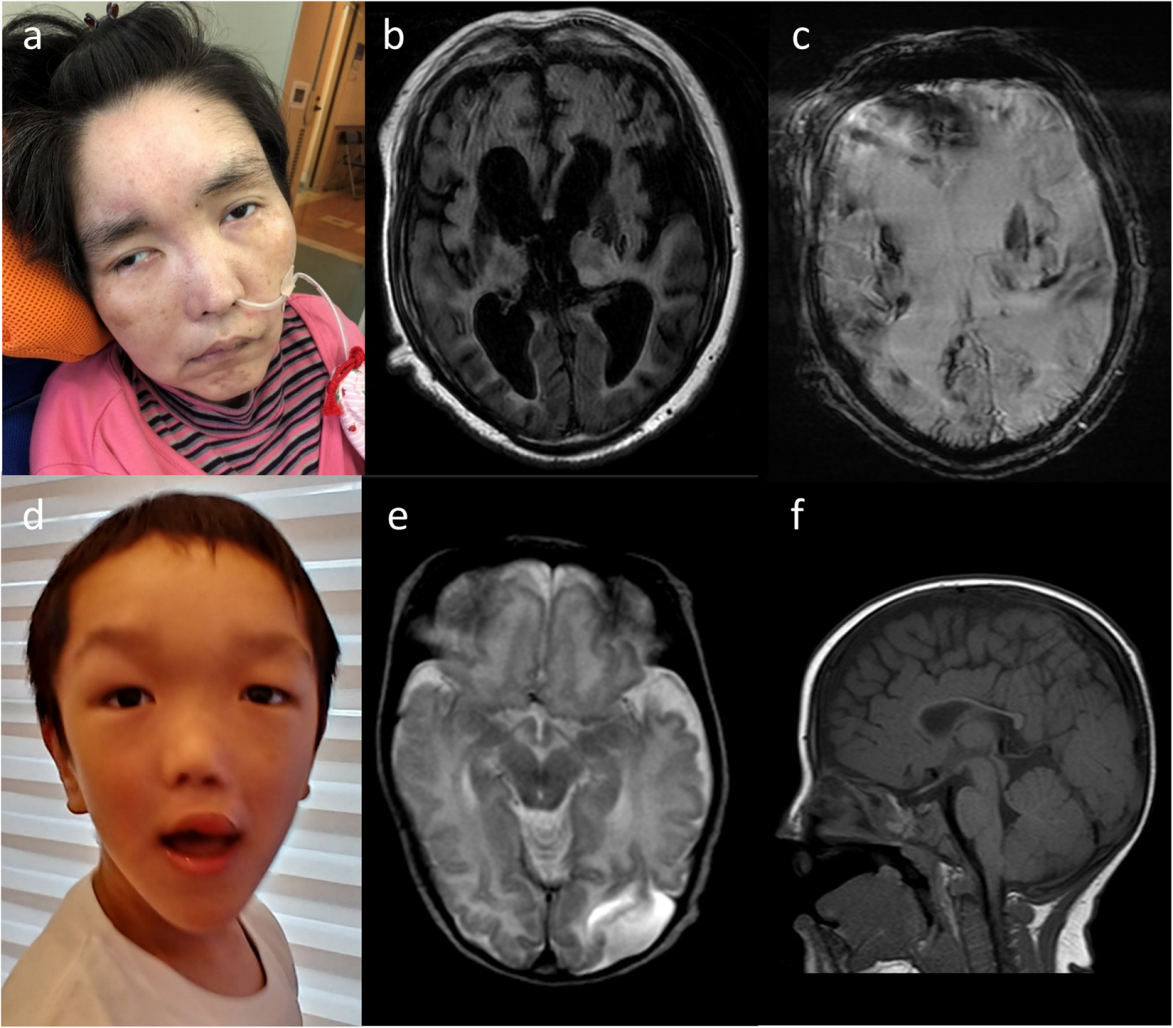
Clinical features of Patients 1 and 2. a A facial photograph of Patient 1 at 39 years of age showing sparse eyebrows and a pointed chin. Brain MRI at 39 years of age. b Fluid-attenuated inversion recovery (FLAIR) imaging showed bilateral enlarged lateral ventricles and reduced white matter volume with high intensity. c T2*-weighted gradient recalled echo imaging showed multiple low-intensity areas in the cerebral cortex and basal ganglia. d A facial photograph of Patient 2 at 6 years of age showing macrocephaly and characteristic facial features, including down-slanted palpebral fissures and a pointed chin. e Brain T2-weighted MRI at 12 days showed subdural hematoma in the left posterior lobe, parenchymal hemorrhage, partial brain atrophy of the left posterior lobe, and reduced white matter volume. f T1-weighted imaging showed thin corpus callosum.
